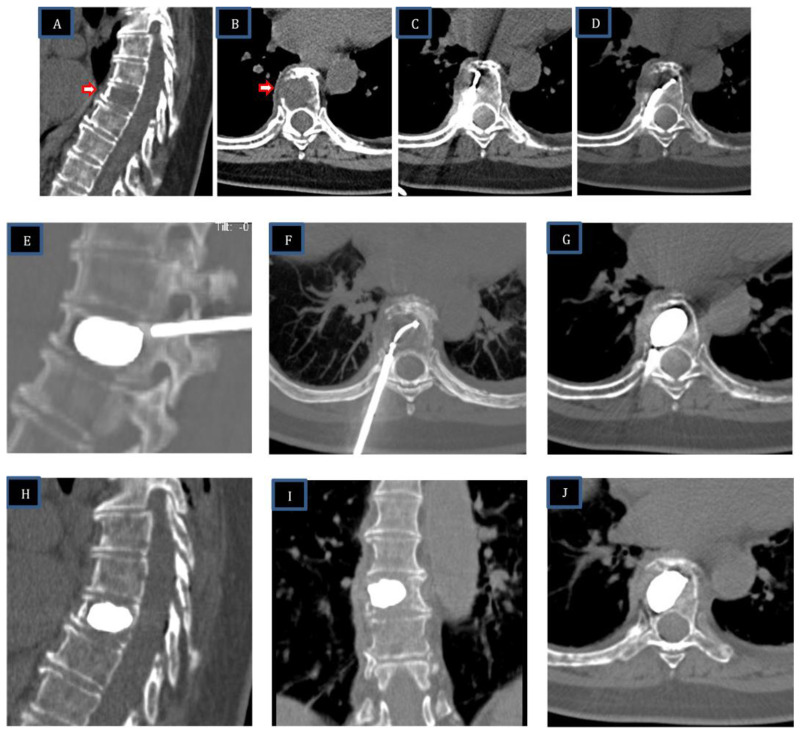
Sixty-year-old female patient with extensive metastatic lytic lesion from breast carcinoma and pathologic fracture of T8. The vertebral body (VB) of T8 has a large interruption of the right lateral wall with initial infiltration of tumor tissue outside VB. (A,B): basal CT—sagittal and axial scans show a large lytic lesion in T8 with cortical right interruption (arrow). (C,D): after channel creation with the steerable PowerCurve^®^ osteotome, two overlapping ablation zones were performed to cover the area of the lytic lesion. The first ablation zone was performed anteriorly with the STAR™ steerable RFA probe in a straight position; the second ablation zone was performed more posteriorly with the steerable RFA probe articulated across the midline. (E–G): Arcadia steerable balloon (SB) placement: deployment of the Arcadia steerable balloon along the axial-coronal plane with pedicle-to-pedicle, endplate-to-endplate inflation, creating a precise void in the ablated tissue. (H–J): Vertebral augmentation—sagittal, coronal, and axial CT scans after StabiliT^®^ high-viscosity cement with controlled hydraulic injection show no cement leakage and reconstruction of vertebral body.
